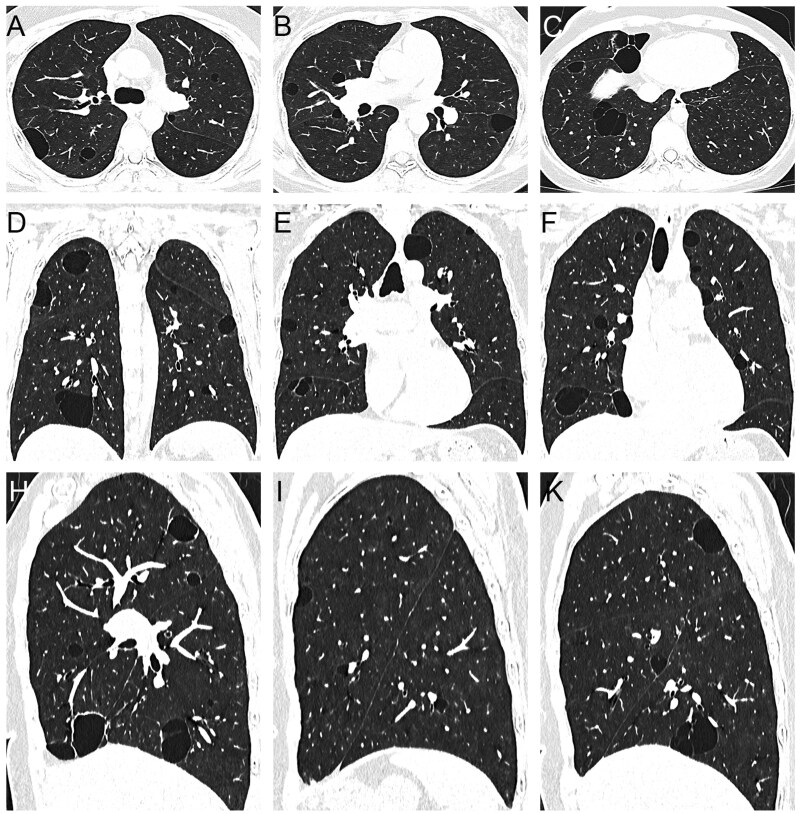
LIP in a 38-year-old woman with Sjögren’s disease. CT images (A-C, axial; D-F, coronal; H-K, sagittal) demonstrate sparse, thin-walled pulmonary cysts of variable shape and size—some septated—clustered in subpleural and peribronchovascular regions of both lungs. Abbreviation: LIP = lymphoid interstitial pneumonia.
